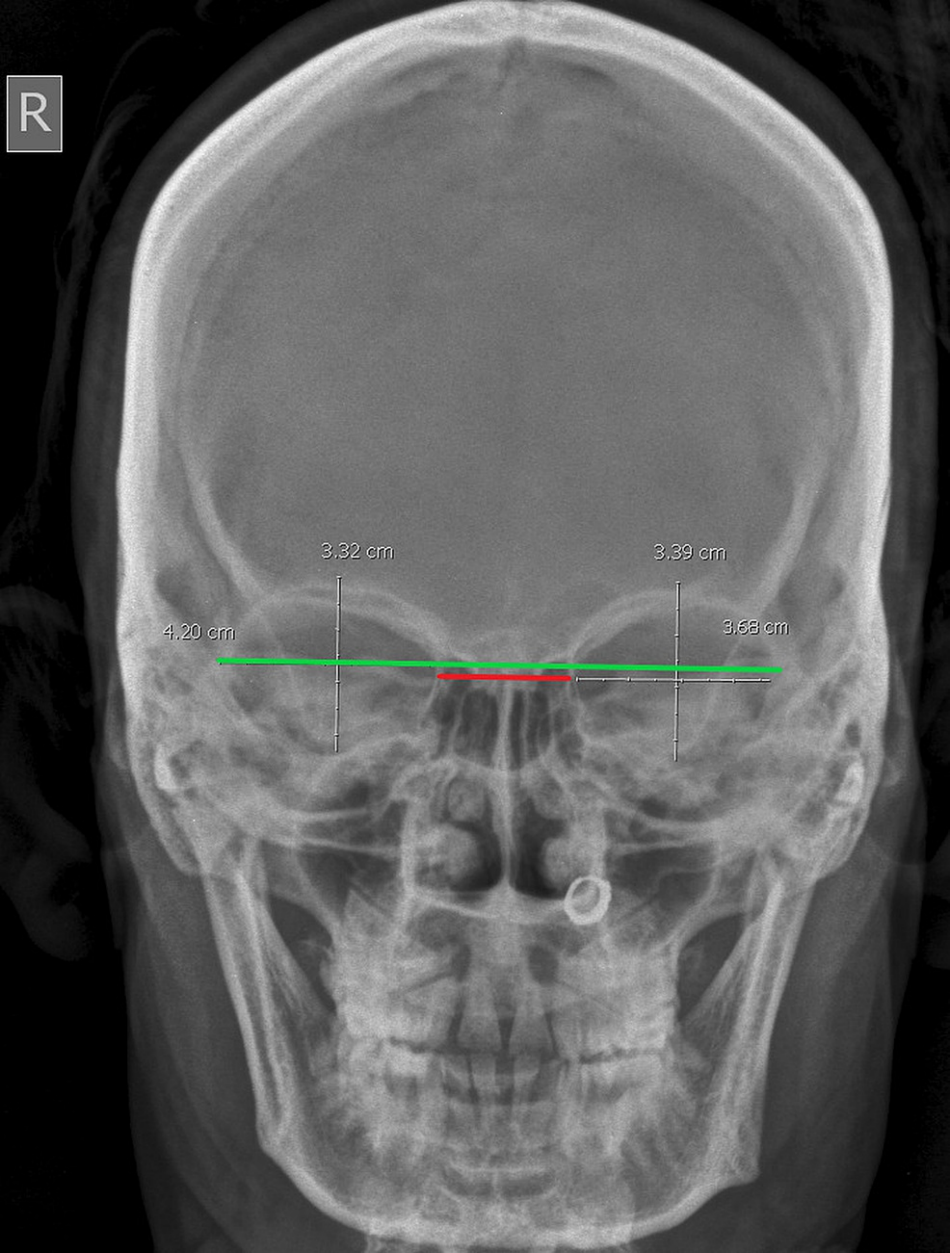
Frontal radiograph showing measurements of interorbital/biorbital distances; red line: interorbital distance; green line: biorbital distance.
